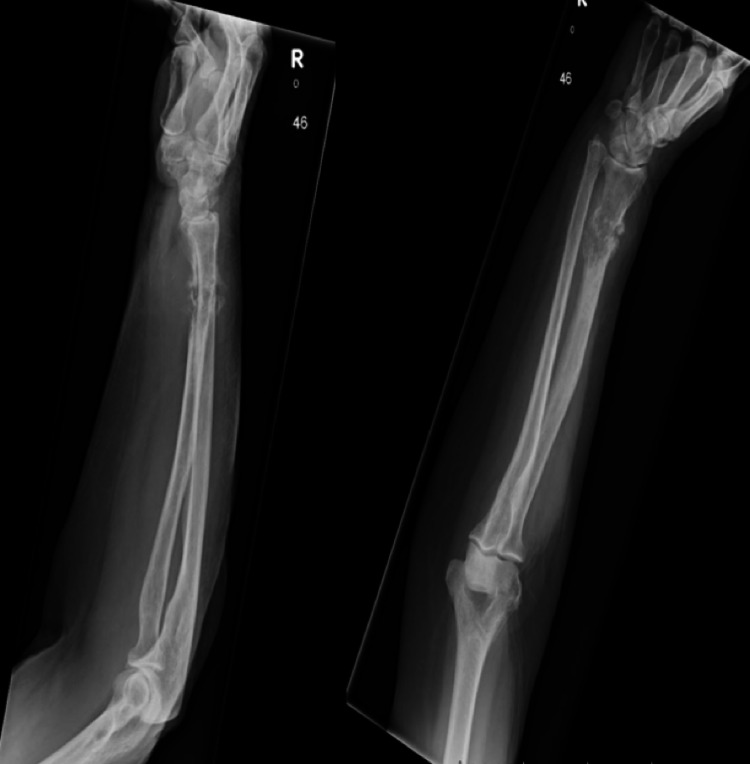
Plain radiographs of right arm Radiographs show a pathological fracture of the distal radius secondary to metastatic melanoma. R: right.
